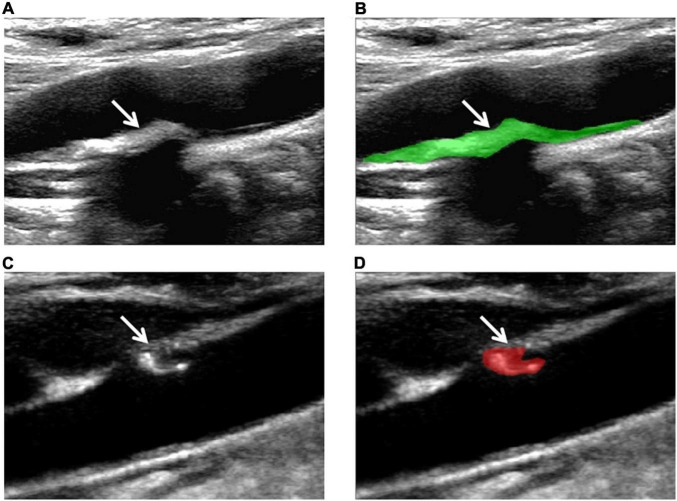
Examples of the manual segmentation in stable and vulnerable plaques. (A) Original ultrasound image of stable plaque (arrow). (B) The segmented area was within the green contour on the largest area on stable plaque (arrow). (C) Original ultrasound image of vulnerable plaque (arrow). (D) The segmented area was within the red contour of the largest area on the vulnerable plaque (arrow).
